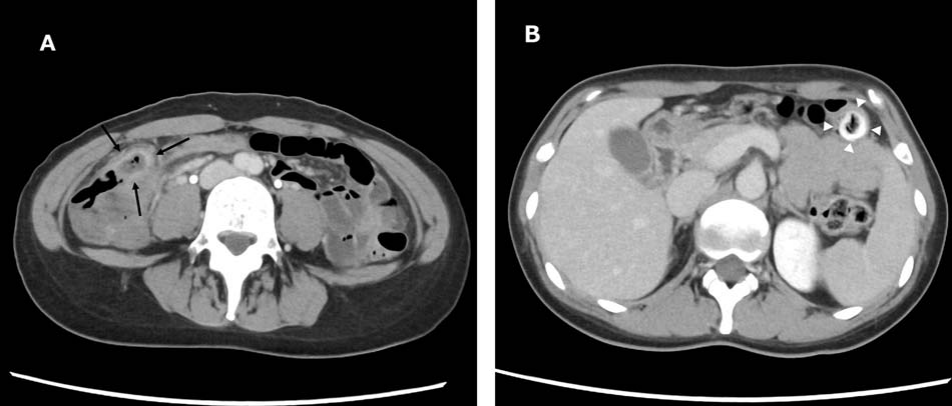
Abdominal and pelvic CT on hospitalization Day 3. Axial images (A and B) showing improved cecal colon diverticulitis (black arrows); however, fecaliths migrated to the transverse colon (white arrowheads).
